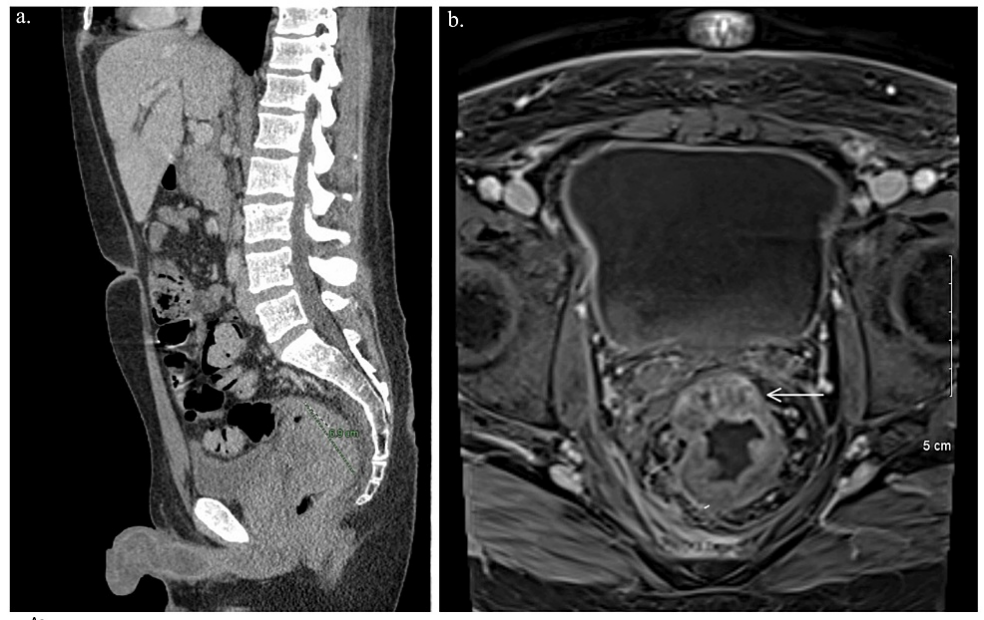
Imaging study results (a) CT abdomen pelvis scan showing constipation with short-segment irregular heterogenous wall thickening of the rectosigmoid colon measuring 6.9 cm (in software notation with dotted line); (b) MRI of the pelvis showed stage cT3b N2a disease with suspicious extra mesorectal lymph node and a defect in the right lateral wall of the rectum, suspicious for contained perforation.
